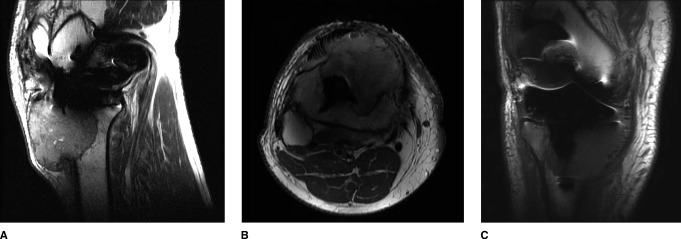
Preoperative MRI of the right knee. Sagittal Proton Dense (PD) (A), axial T1 Fat Supressed (FS) (B), and coronal T1 FS (C). Imaging demonstrates an erosive, well-circumscribed lesion extending from the infrapatellar fat pad into the proximal tibia metadiaphysis.
